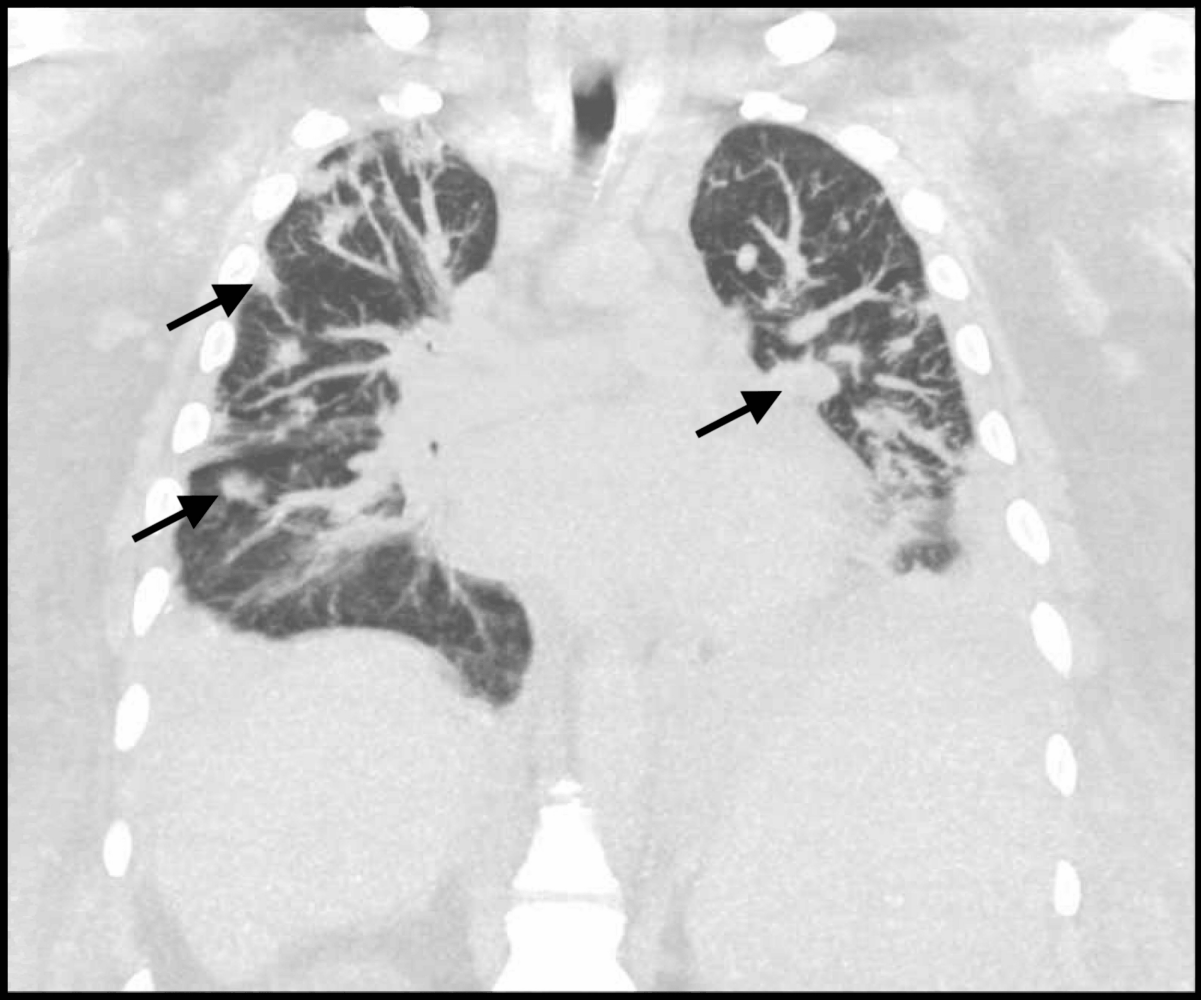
CT lung showing multinodular opacities suggestive of necrotizing pneumonia and abscess formation (black arrows)
